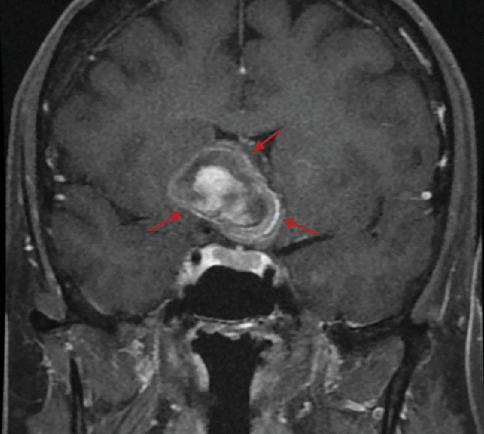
MRI, coronal T1 image showing a suprasellar mass with contrast enhancement consistent with active high flow into the lumen of a right supraclinoid carotid artery aneurysm. There is surrounding mass effect with compression of the right and left optic nerves and anterior chiasm with greater compression of the right optic nerve versus the left optic nerve.
